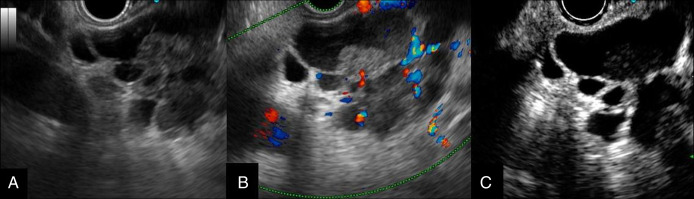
(A) EUS demonstrated a high-echoic area within the cyst. (B) Color Doppler imaging showed no blood flow within the high-echoic area. (C) Contrast-enhanced EUS using Sonazoid revealed no enhancement in the high-echoic area, suggesting intracystic hemorrhage. EUS, endoscopic ultrasound.
